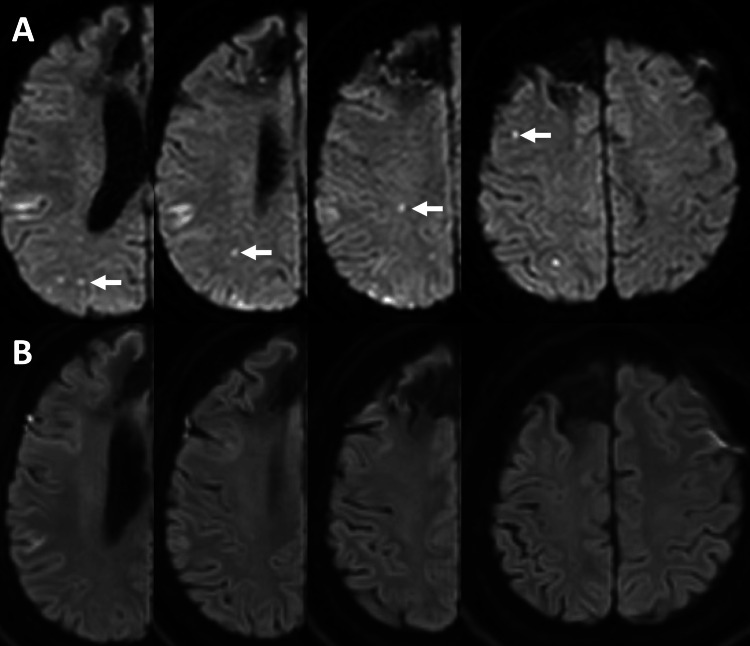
Postoperative diffusion-weighted magnetic resonance imaging (DWI) (A) DWI on postoperative day seven shows multiple punctate high-intensity lesions in the right cerebral hemisphere. Arrows indicate representative lesions; (B) Follow-up DWI on day 14 shows no new ischemic lesions
